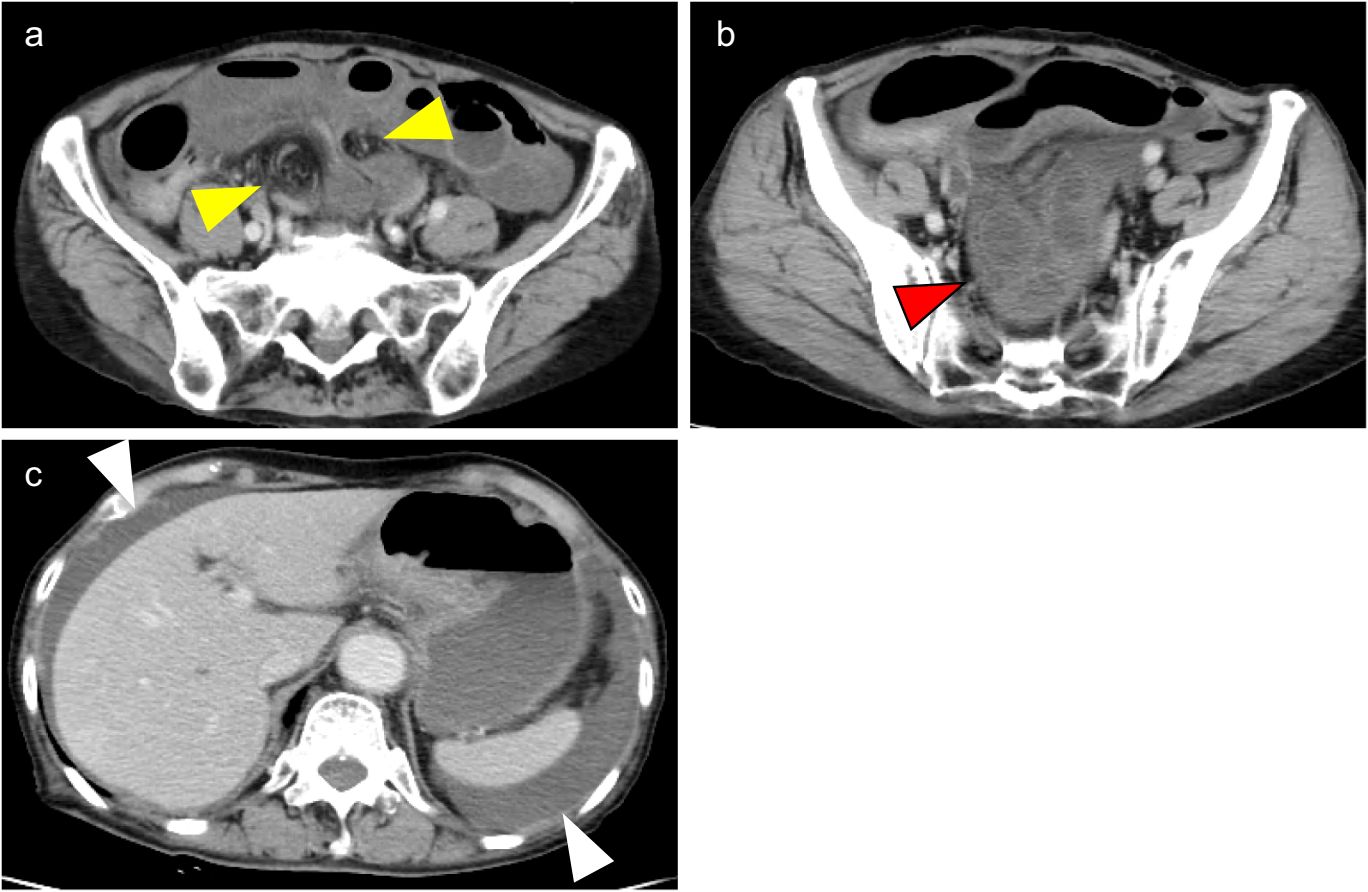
Contrast-enhanced CT imaging. a Hernia portal where the small bowel enters forming a closed loop (yellow arrow) b Dilated small bowel with poor contrast effect (red arrow) c Ascites (white arrow)
